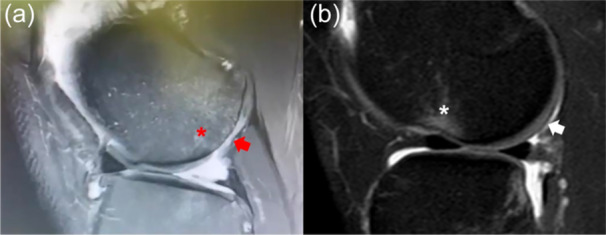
Sagittal MRI showing the subchondral bone oedema (red asterisk) and cartilage loss (red arrow) in the posterior area of the LFC in a 26‐year‐old patient who sustained subtotal lateral meniscectomy 8 years before (a). Sagittal MRI of an acute ACL tear, with signs of traumatic subchondral bone oedema in the anterior portion of the LFC (white asterisk) and intact cartilage in the posterior part (white arrow) (b). ACL, anterior cruciate ligament; LFC, lateral femoral condyle; MRI, magnetic resonance imaging.
